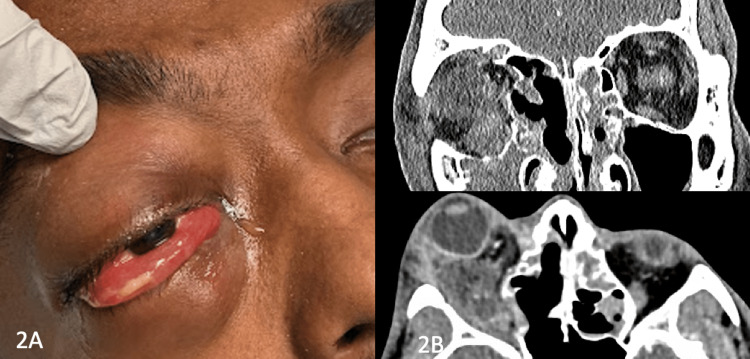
Second presentation - 2A. Photograph of the right eye. 2B. CT scan coronal and axial views demonstrating worsened orbital inflammation/infection.
